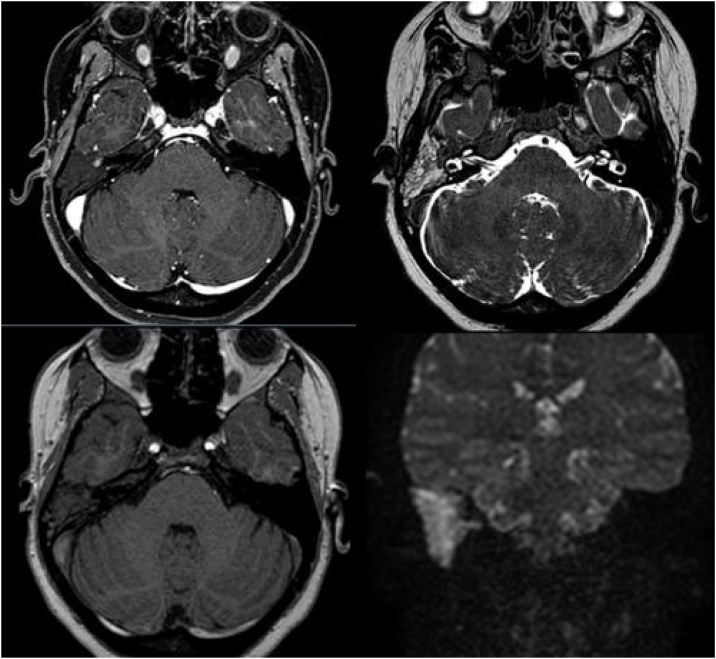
MRI demonstrating an iso-intense mass filling the mesotympanum, in contact with the internal carotid artery, with no evidence of bone erosion.
